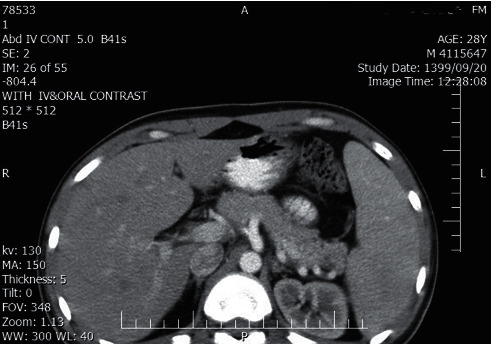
The splenic infarction resolves entirely in the following abdominal CT scan with IV contrast.
